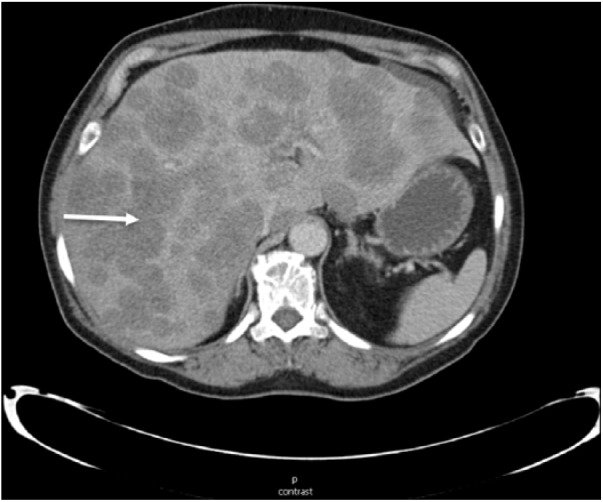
Computed tomography scan of the abdomen suggesting diffuse hypodense liver lesions (arrow) suggestive of metastatic disease.
